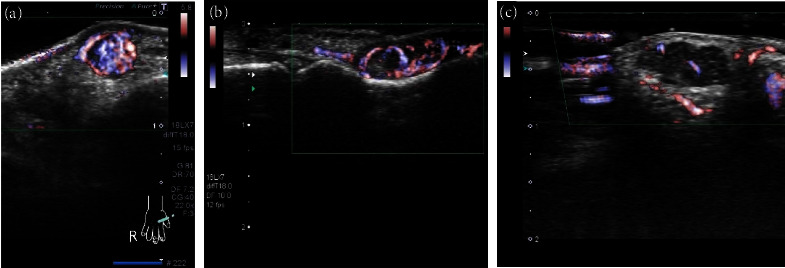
Ultrasound images of blood-flow patterns: (a) high blood flow, (b) moderate blood flow, and (c) low blood flow.
